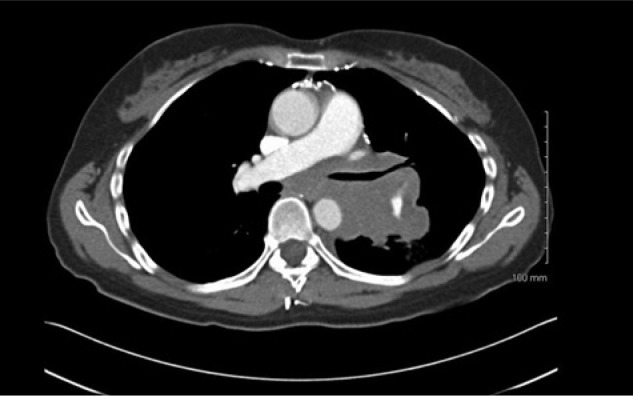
CT scan showing the infiltrating mediastinal mass.
